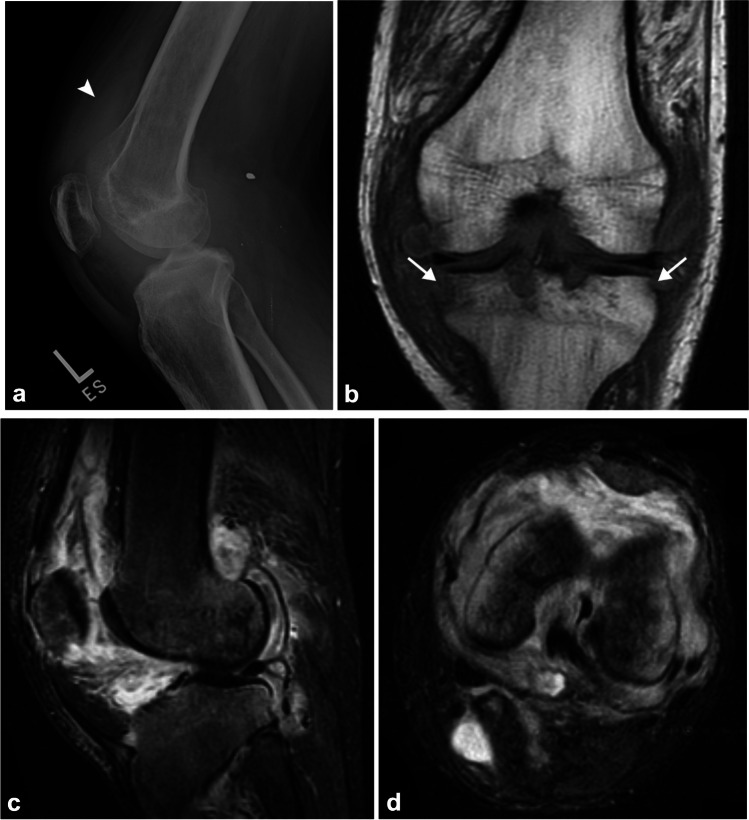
Thirty-eight-year-old man with right knee pain and swelling. Lateral radiograph (a) and Cor T1 (b), Sag STIR (c), and axial STIR (d) MR images of the right knee demonstrate juxta-articular osteopenia with a peripheral erosion (*arrow*) and relative preservation of the joint space with only gradual narrowing over a period of five months (Phemister’s triad) and a large joint effusion (*arrowhead*) with extensive synovitis on MRI. Joint aspiration demonstrated acid-fast bacilli, and cultures grew *Mycobacterium tuberculosis*, diagnostic for tuberculous arthritis
